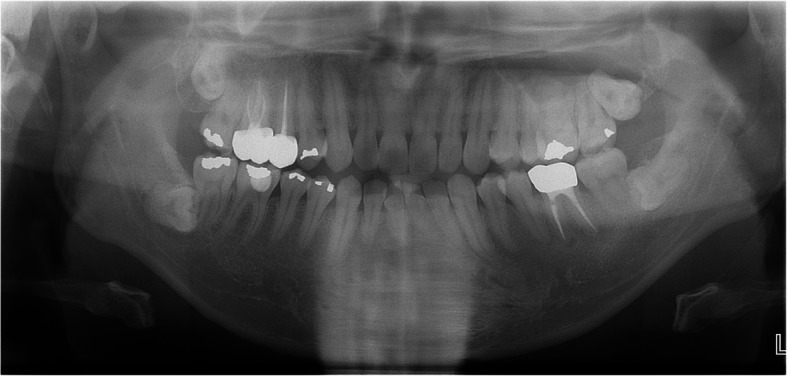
The panoramic radiograph of a 32-year-old male patient with odontogenic keratocyst (OKC) involving an impacted left upper third molar
